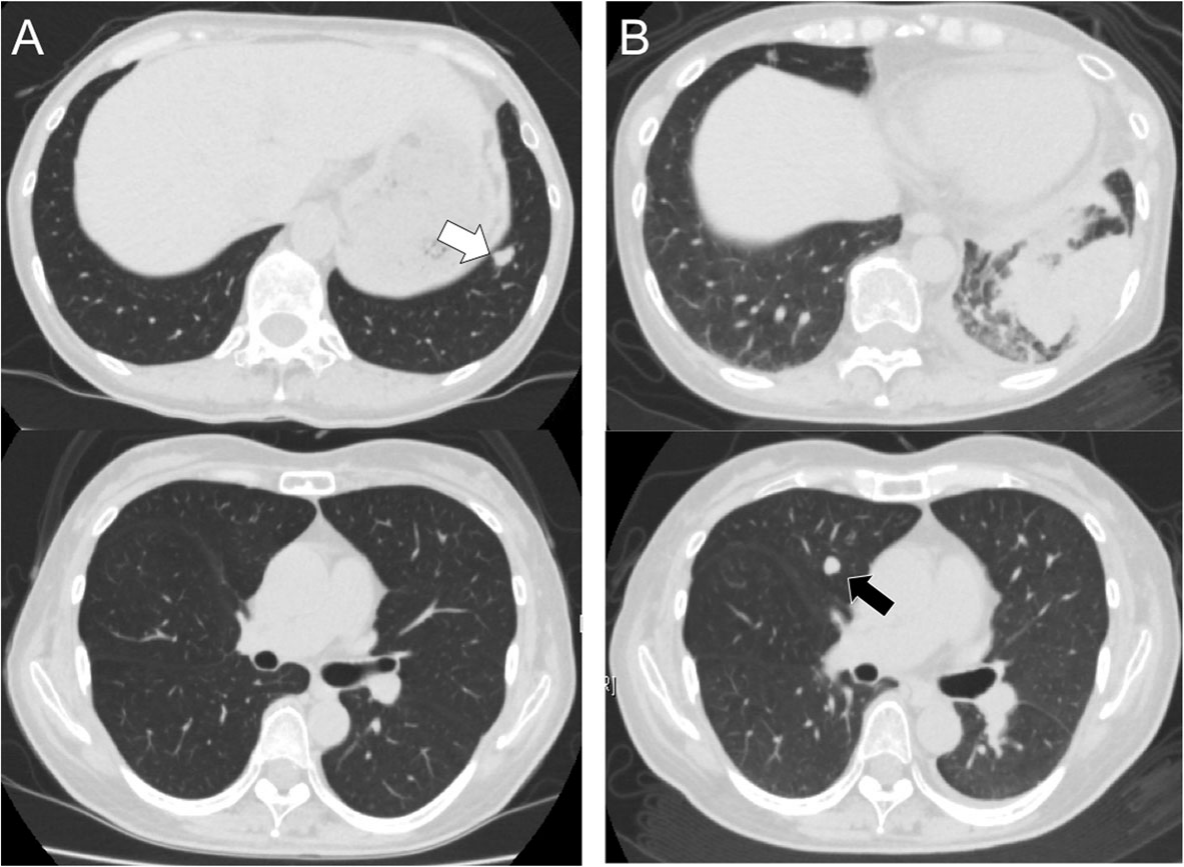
a Chest computed tomography (CT) performed 1 month before admission showed a pulmonary nodule in the left lower lobe (white arrow). b Chest CT on admission showed consolidations in the same lobe, and a new pulmonary nodule in the right middle lobe (black arrow)
